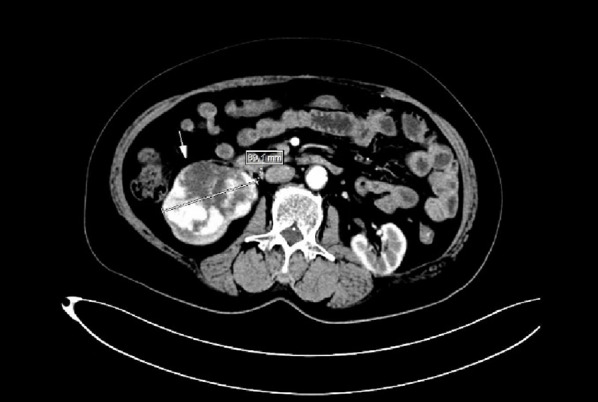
CT scan reveals an 8.9 cm × 6.2 cm mass in the lower pole of the right kidney involving the renal pelvis
